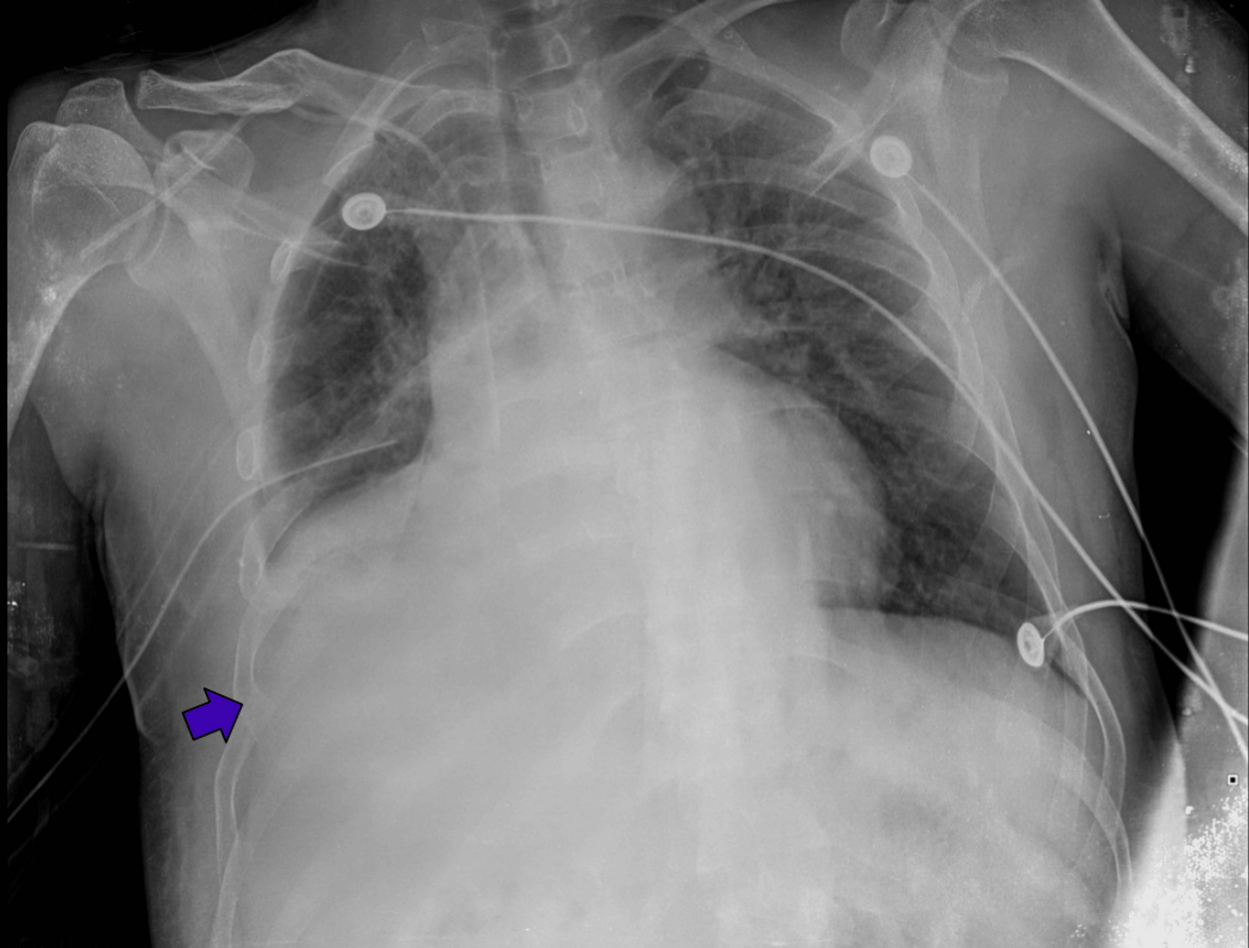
First posteroanterior (PA) chest X-ray on admission. Despite the rotation of the X-ray, right pleural effusion with poor aeration and increased lung density (blue arrow) is appreciated, likely due to contusion or edema. A jugular catheter with the tip in the right atrium and intercostal tube at the lung base and the right rib fractures from the fifth to the 12th rib were noted.
